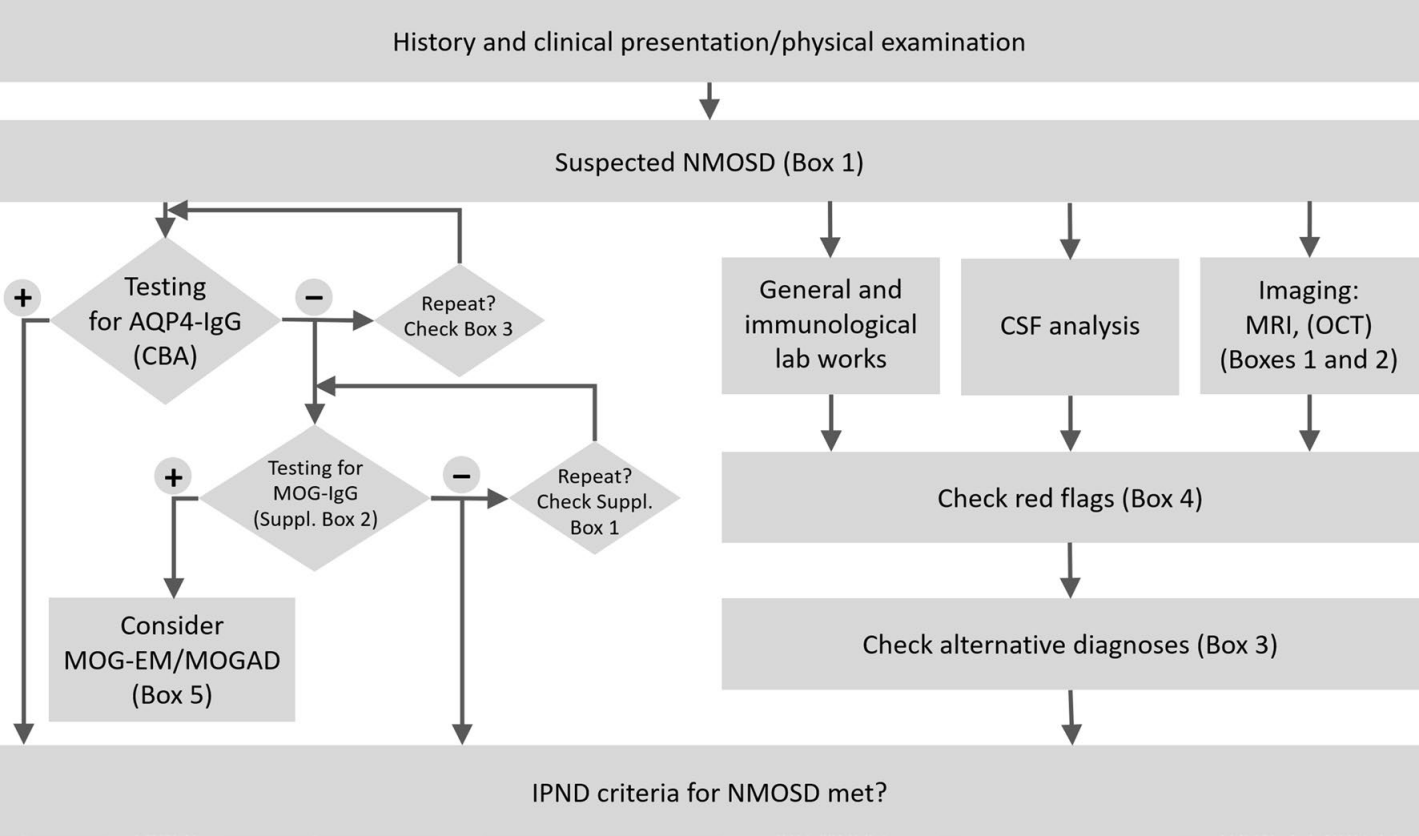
Proposed algorithm for the diagnosis of NMOSD. *AQP4-IgG* aquaporin-4 immunoglobulin G antibodies, *CSF* cerebrospinal fluid, *MOG-EM/MOGAD* myelin oligodendrocyte glycoprotein (MOG) antibody-associated encephalomyelitis/MOG autoantibody-associated disease, *MRI* magnetic resonance imaging, *OCT* optic coherence tomography
